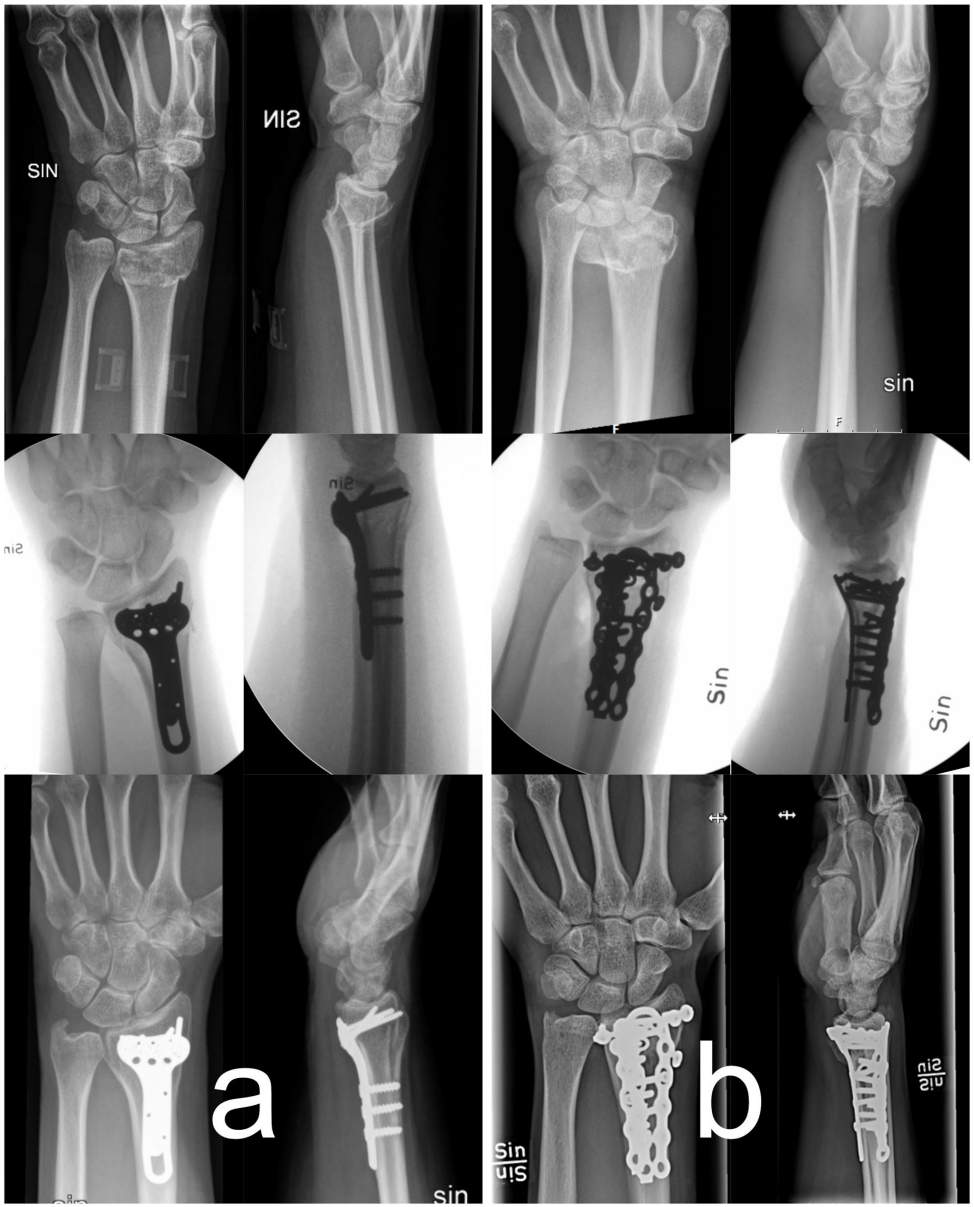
Radiographs preoperative, interoperative, and at 12-moths follow-up. a volar plating, b combined plating
